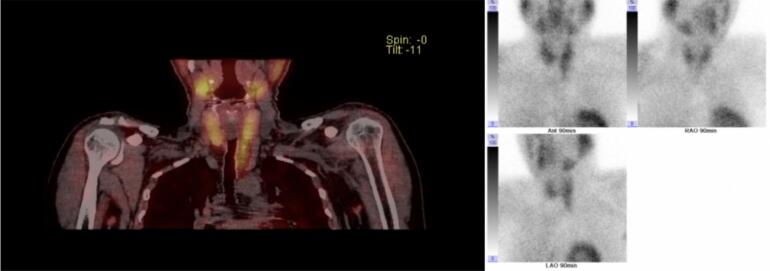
Sestamibi imaging: increased FDG uptake identified near the left inferior pole of the thyroid, extending inferiorly.
